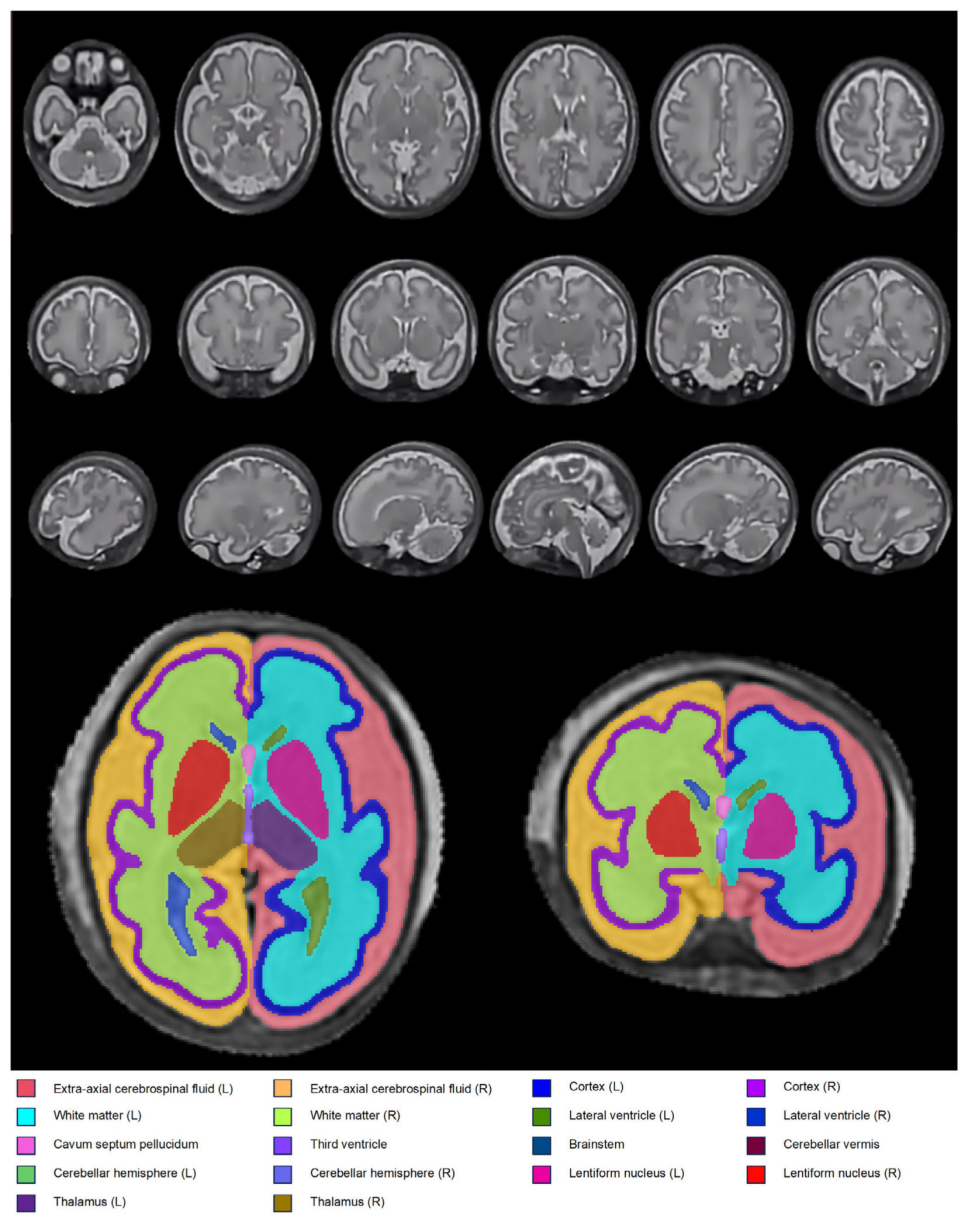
Example 3D slice-to-volume reconstructions of T2-weighted fetal brain MR images in coronal (first row), axial (second row), and sagittal (third row) views. These images were acquired at a gestational age of 34^+0^ weeks. Regional segmentations, based on the developing Human Connectome Project atlas segmentation protocol with 19 label regions of interest, generated by BOUNTI automated fetal brain MRI segmentation tool are shown in axial (bottom left) and coronal (bottom right) slices.
